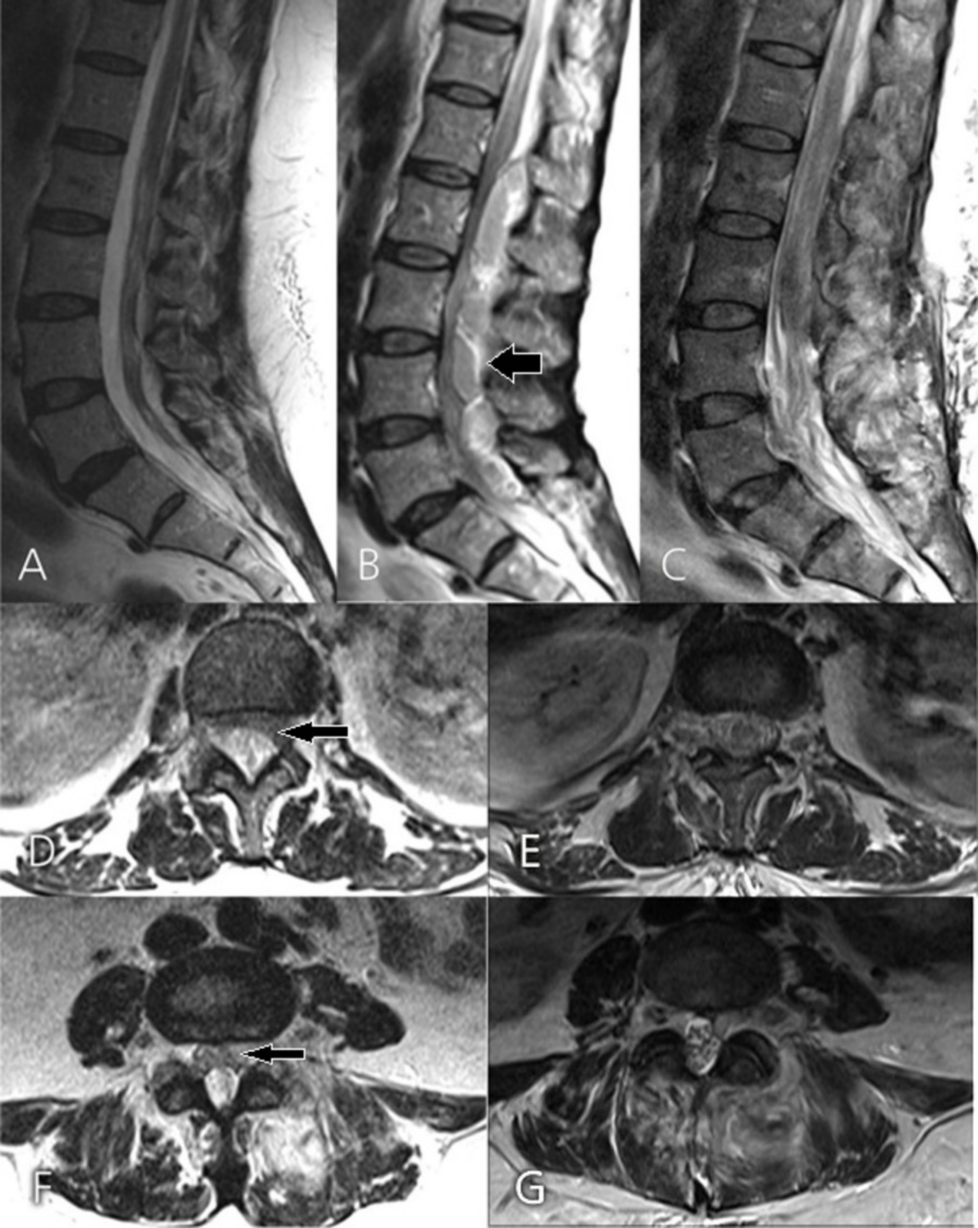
Comparative pre- and postoperative MRI images of Case #1 Comparative sagittal T2-weighted MRI at 2 months (A) and 15 months (B) after the initial presentation, and one day after surgery (C). B: Epidural abscess extending from L1 through S1 (arrow). C: Postoperative image demonstrating complete abscess evacuation. Axial T2-weighted MRI at L1-2 (D) and L4-5 (F) taken 15 months after the initial presentation (arrow representing the epidural abscess) and corresponding axial cuts after surgery (E and G).
